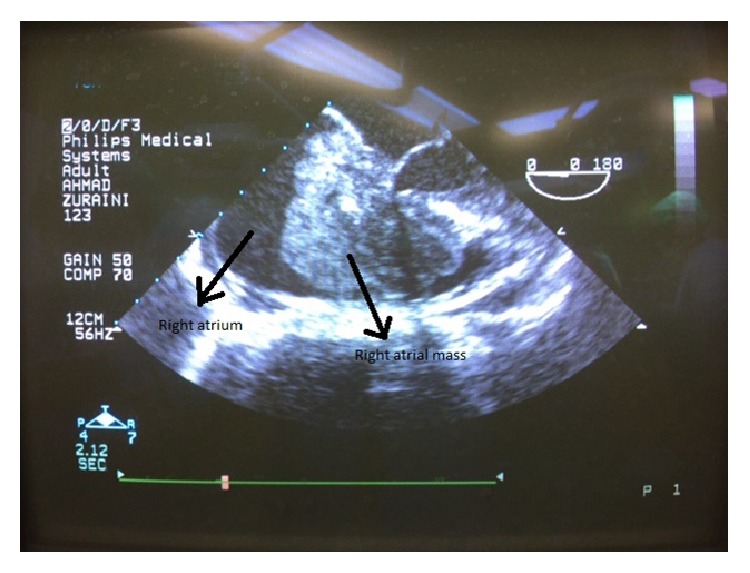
Intraop. transechocardiograph shows right atrial mass attached to interatrial septum.
